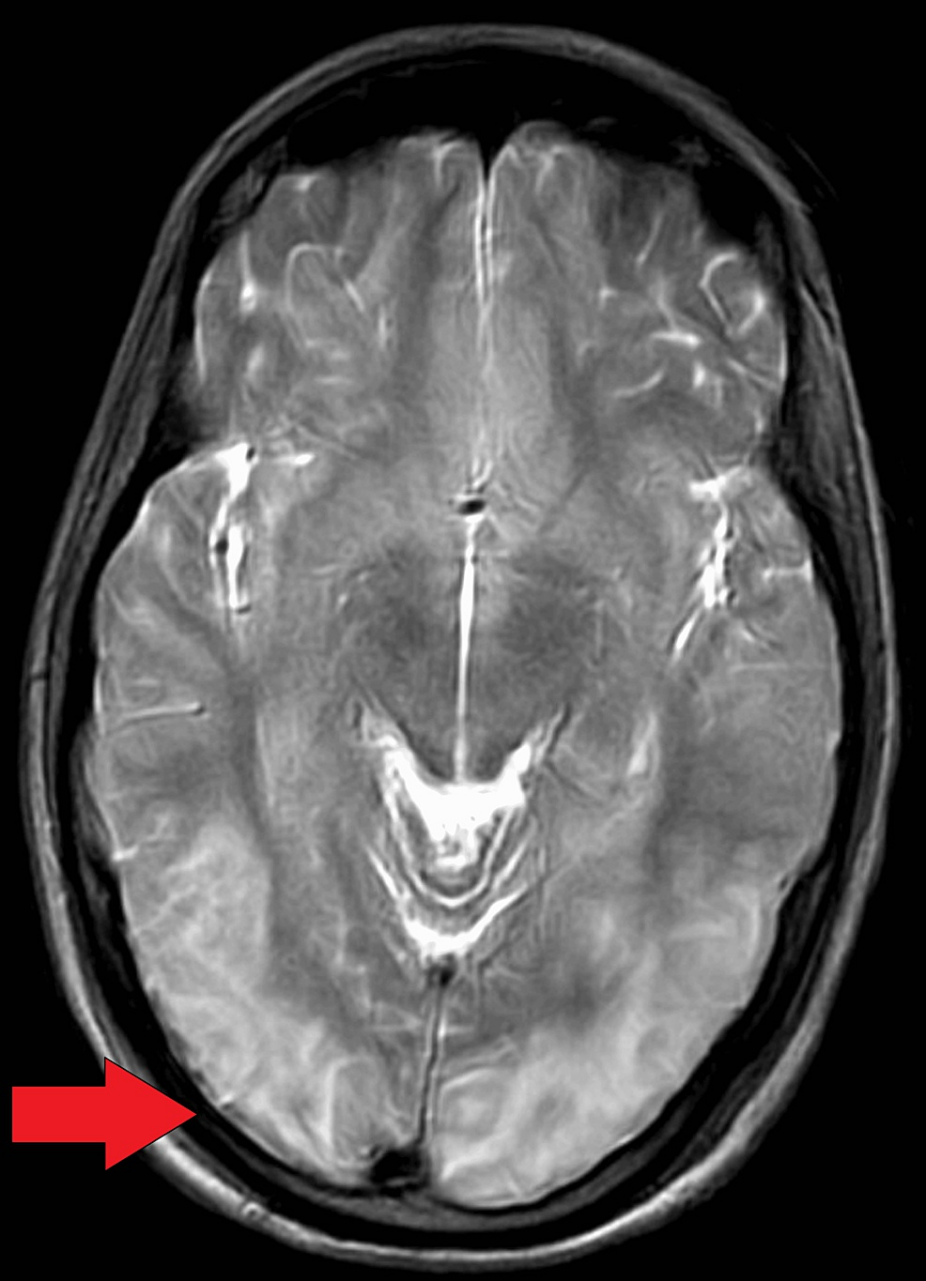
Abnormal hyperintense lesions confluent in both parieto-occipital lobes on T2-weighted MRI scan.
